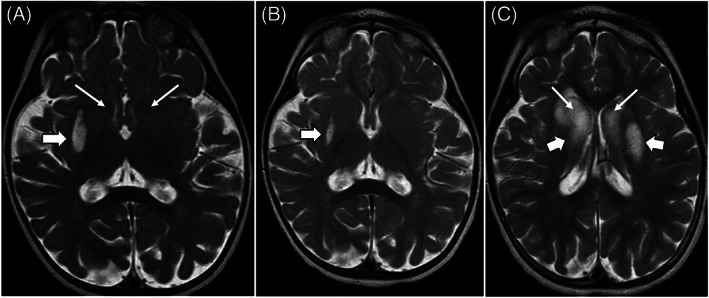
(A) T2 axial MRI of the Brain from 11 months of age. An edematous lesion is present within the right putamen nucleus (large arrow). The caudate nuclei remain radiographically unaffected (thin arrows). (B) T2 axial MRI of the brain at 15 months of age showing the formerly edematous right‐sided lesion of the putamen evolving into cystic cavitation (thick arrow). (C) T2 axial MRI of the brain at 2 years, 5 months of age showing massive edematous changes of the caudate nuclei (thin arrows), as well as of the putamen nuclei bilaterally (thick arrows)
